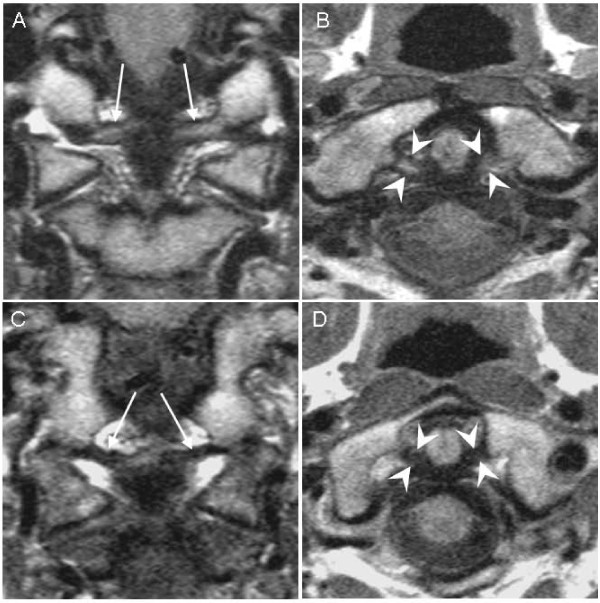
MRI of alar and transverse ligaments. High-resolution proton-weighted MRI sections of upper neck alar and transverse ligaments. Grade 3 alar ligament high-signal changes (arrows) on coronal section (A) and grade 2 transverse ligament high-signal changes (arrowheads) on axial section (B) in two patients recovered (NDI ≤ 8%) at follow-up. Grade 0 alar ligaments (arrows) on coronal section (C) and grade 0 transverse ligament (arrowheads) on axial section (D) in two different patients reporting disability (NDI > 8%) at follow-up for comparison.
